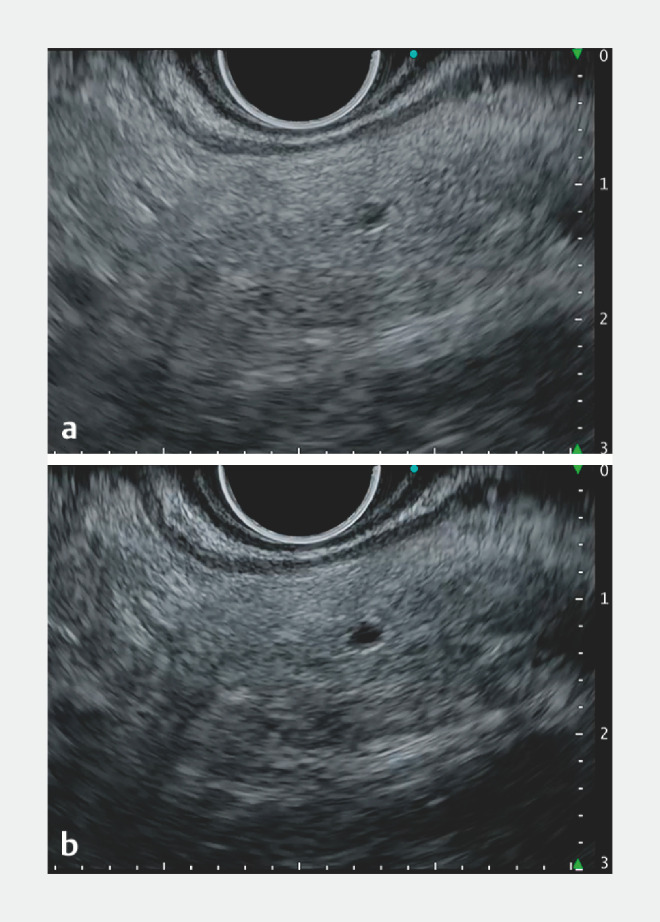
Endoscopic ultrasound (EUS) images of the upper stream of the narrow pancreatic duct (1.2 mm in diameter) during EUS-guided pancreatic duct drainage. Low echo reduction (LER) enhances the contrast by suppressing the low echo areas without overemphasizing high echo areas. The LER ranges from Lv.1 to 20 and is usually set at Lv.3.
